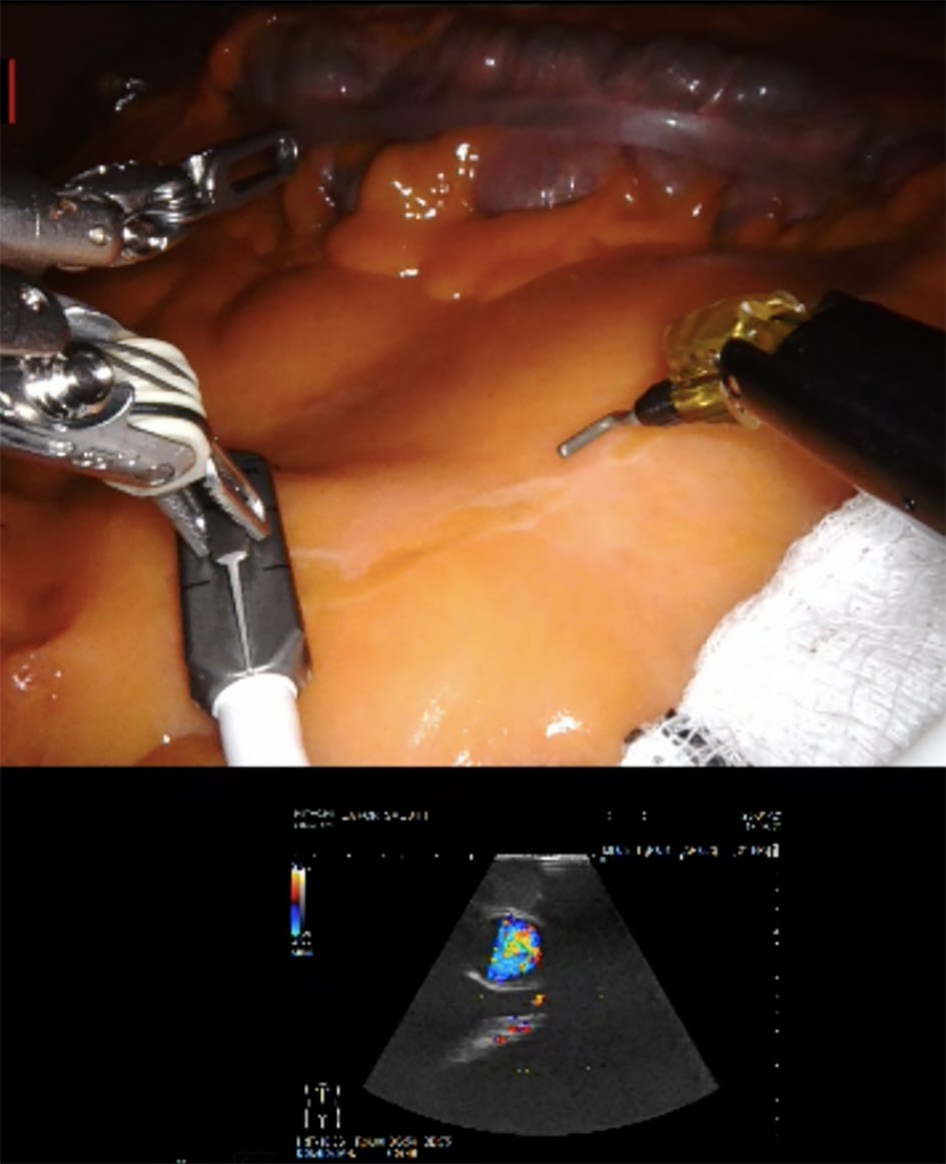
The ultrasound view in TilePro TM vision at the surgeon’s console and marking of the SMV as identified by the ultrasound device using monopolar diathermy with hook
